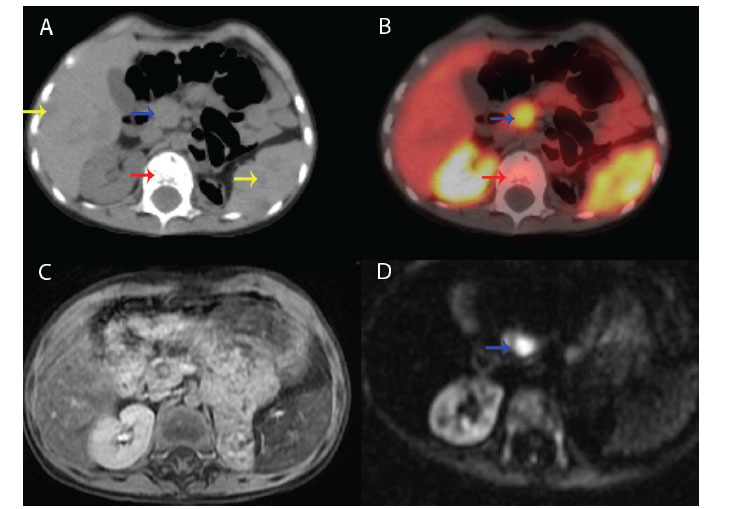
An uncinate process of the pancreas exhibits intense uptake, as seen in the CT image (A) and the Ga68-DOTATATE fusion image (B) of Case 2. T2-weighted (C) and diffusion-weighted images (D) also demonstrate the presence of a tumor that is causing diffusion restriction (blue arrows). Additionally, a small focus is seen on the organ's tail. The clinician was informed of multiple bone infiltrations (*i.e*., T12 vertebrae-red arrows) and liver/spleen metastases (yellow arrows) in addition to pancreatic involvement.
